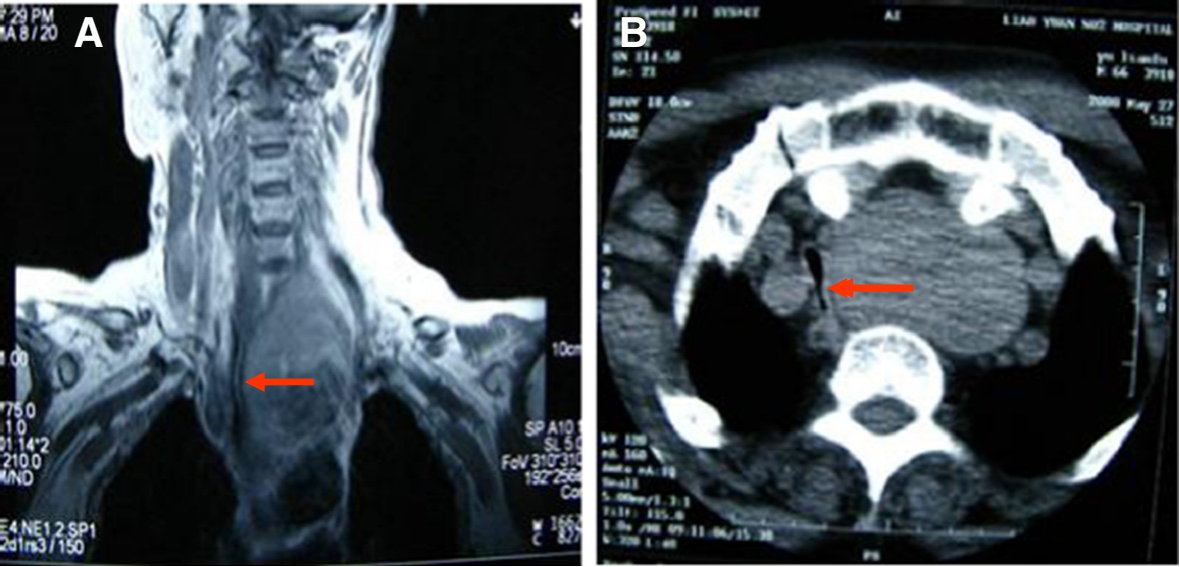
Magnetic resonance imaging (MRI) (A) and computed tomography (CT) (B) demonstrated a massive elliptical substernal goiter, displacing the trachea and adjacent large vessels to the patient’s right, contributing to severe intrathoracic tracheal compression up to 6 cm in length and the narrowest caliber of the trachea (arrows in light red) was about 3.0 mm in diameter.
